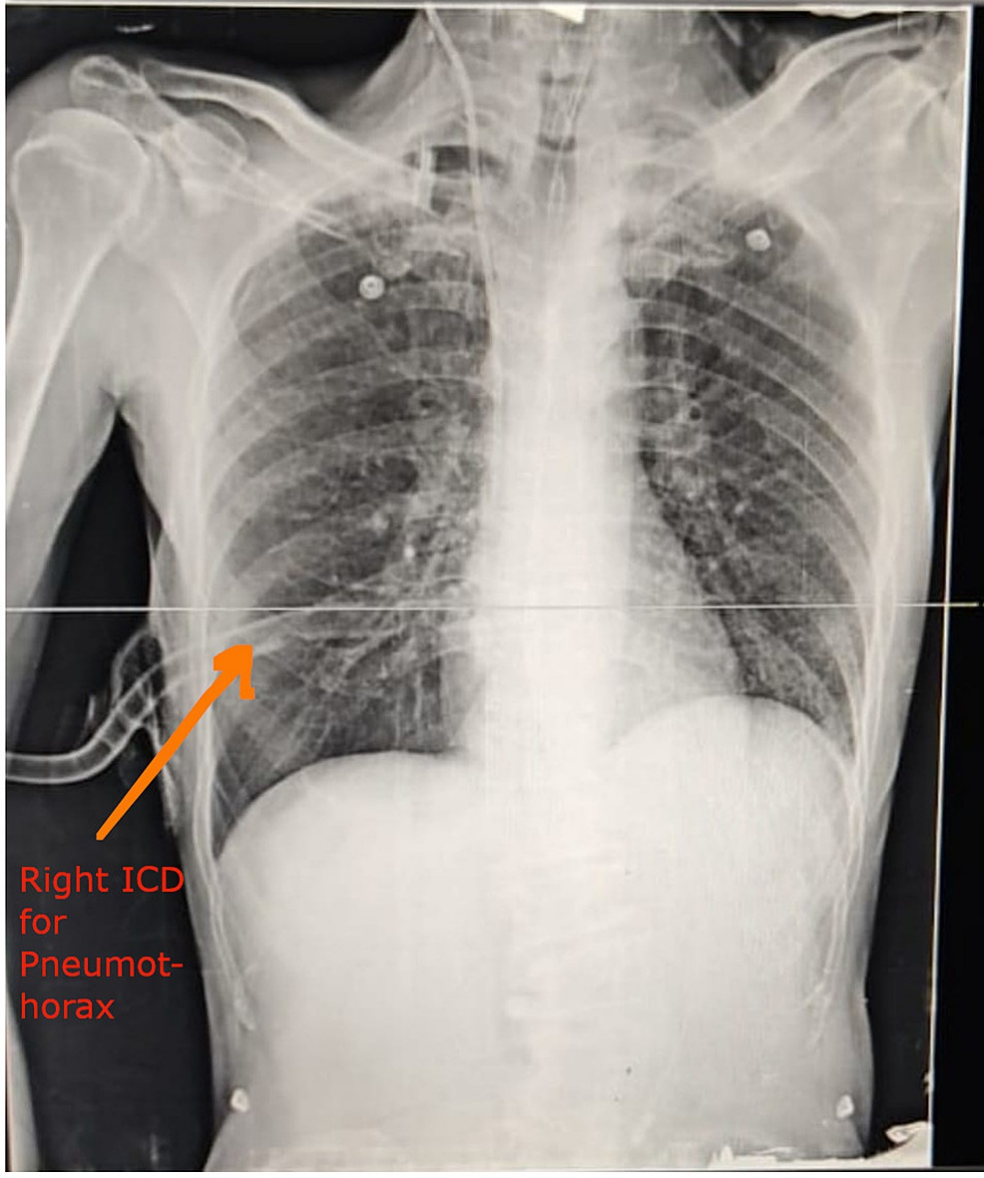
Chest radiography showing right intercostal drainage tube in situ for pneumothorax (Case 1) ICD: Intercostal drainage tube
